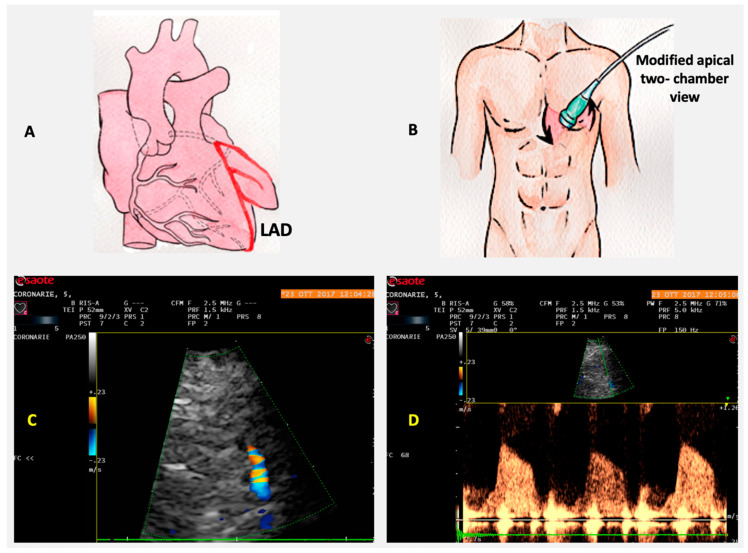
Coronary flow analysis. The distal left anterior descending (LAD) tract is more suitable to investigate coronary microvascular function because it is between large epicardial arteries and microvasculature (A). The acoustic window is, in the left decubitus position, around the midclavicular line in the fourth or fifth intercostal space (B). After detecting by color the LAD image (C), coronary flow by Doppler is represented by a biphasic wave, with a lower peak during systole and a higher peak during diastole, for the effect of myocardial contraction (D).
